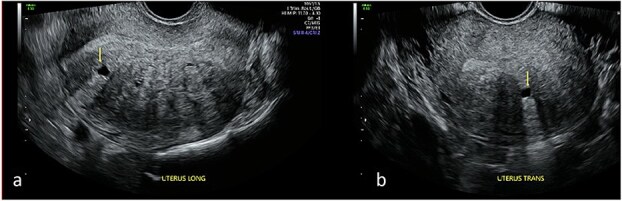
Transvaginal ultrasound of the uterus demonstrating adenomyotic spaces in the myometrium and sub-endometrial border in longitudinal (a) and transverse planes (b), with a notable absence of decidual reaction around the cystic spaces.
